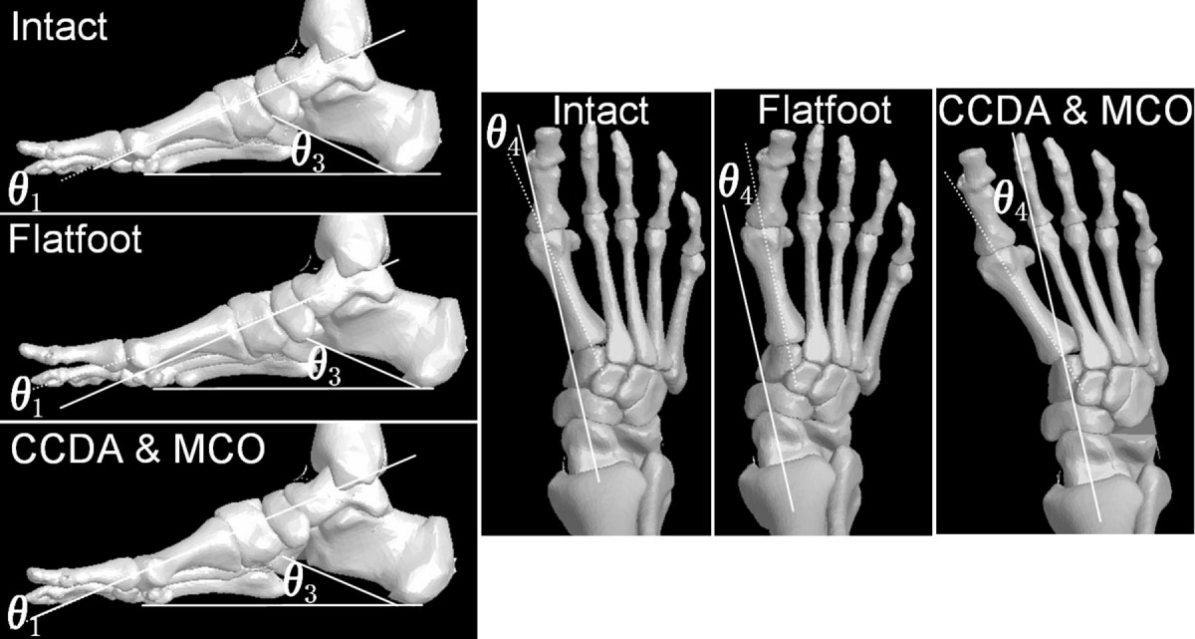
Radiographic views of the intact, flatfoot, and flatfoot treated with CCDA and MCO to highlight some of the angular changes resulting from surgical correction. Lateral views show representations of L-T1MT angle, *θ*_1_ and L-CP, *θ*_3_ (left series); AP views include a representation of the AP-T1MT angle, *θ*_4_ (right series). Note large changes in AP forefoot ab/adduction and subtle changes laterally in medial column alignment and height.
